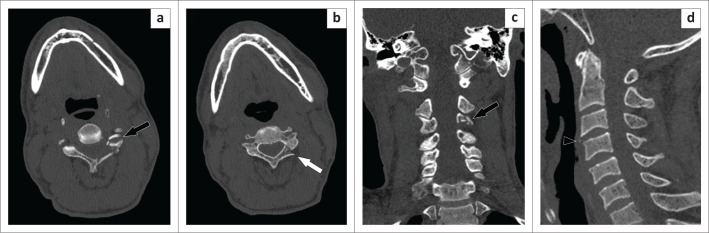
Axial (a and b) and coronal (c) reconstructions of the cervical spine CT showing comminuted fractures of the left superior and inferior facets of C4 (black arrow) and left lamina of C4 (white arrow). Sagittal (d) reconstruction shows a small avulsion fracture of the anterosuperior margin of the C4 vertebral body (black arrow). These fractures represent an unstable cervical spine injury.
